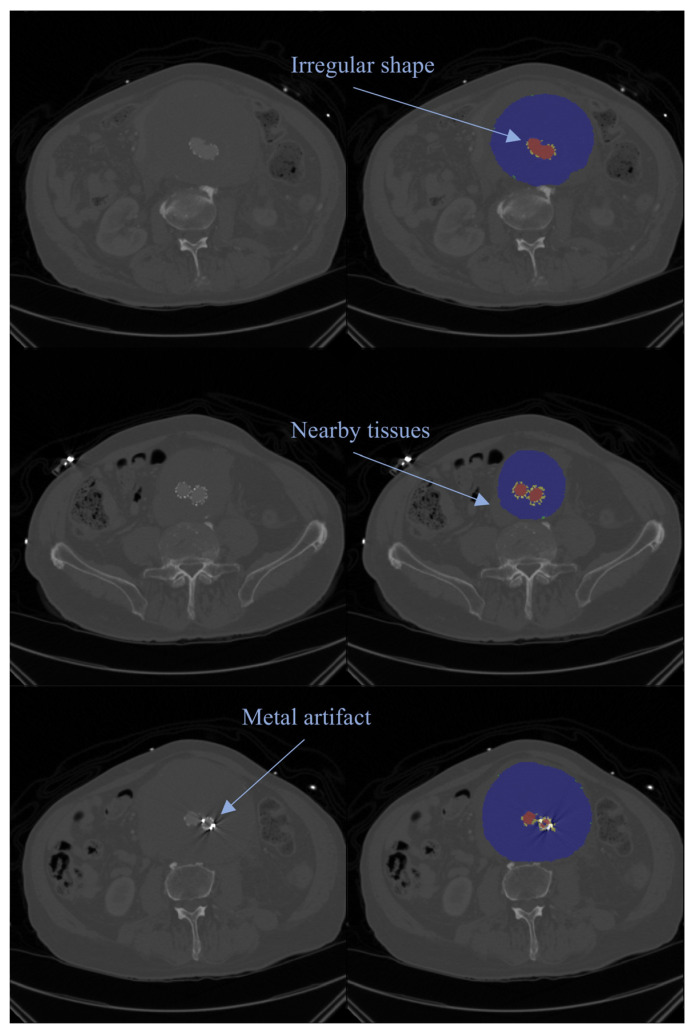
Representative postoperative CTA images showing axial slices. Left column: original CTA images; right column: corresponding manual segmentations (red: lumen; blue: ILT; yellow: stent grafts). The arrows highlight major challenges encountered in ILT delineation, including irregular thrombus morphology, interference from nearby tissues, and artifacts caused by metallic implants, all of which can compromise segmentation accuracy.
